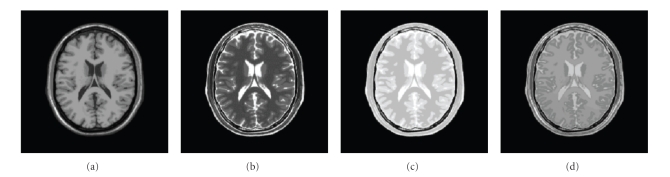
From left to right: synthetic noise free T1, T2, and PD (low and high flip angle) weighted MR images.
